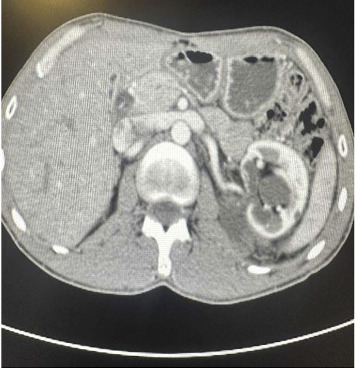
Subsequent computed tomography after one year revealing quasi-normal aspect of the kidneys.
